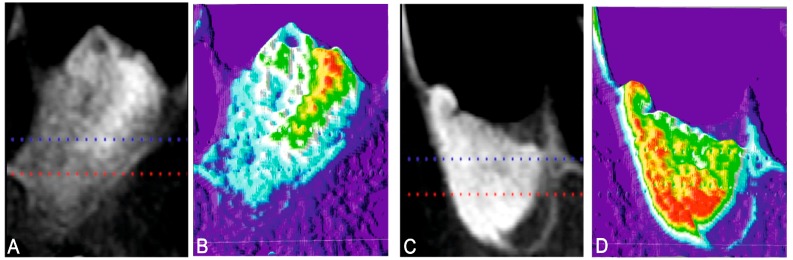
X-ray of the implant site six months after xenograft materials implantation and the corresponding color bone density (A,B) the PBM material and (C,D) the BPM material.
